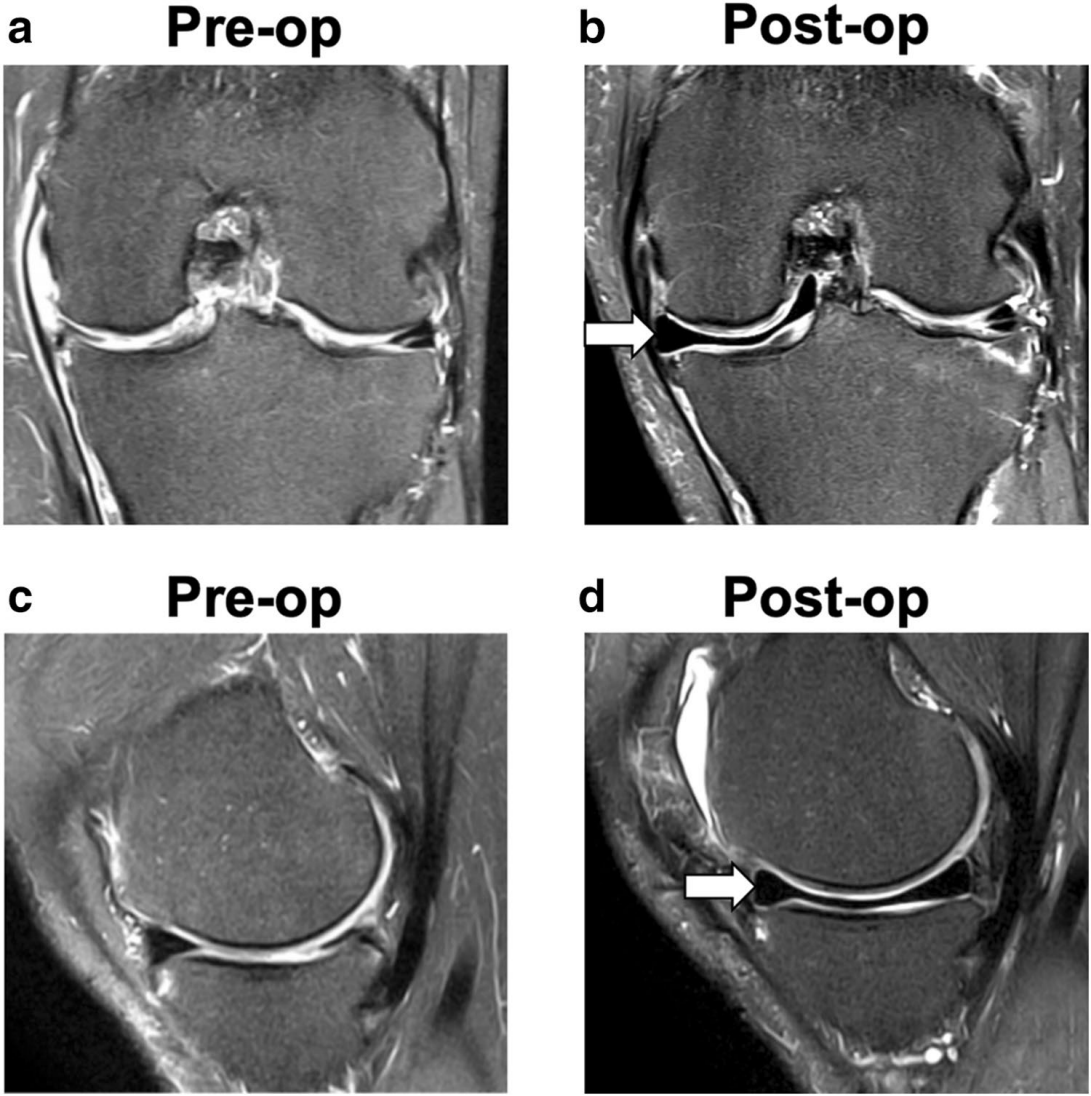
Magnetic resonance imaging (MRI) of a knee before (pre-op; a and c) and after (post-op; b and d) implantation of the MMR implant from the coronal (a, b) and sagittal (c, d) views. Arrows indicate the MMR implant
